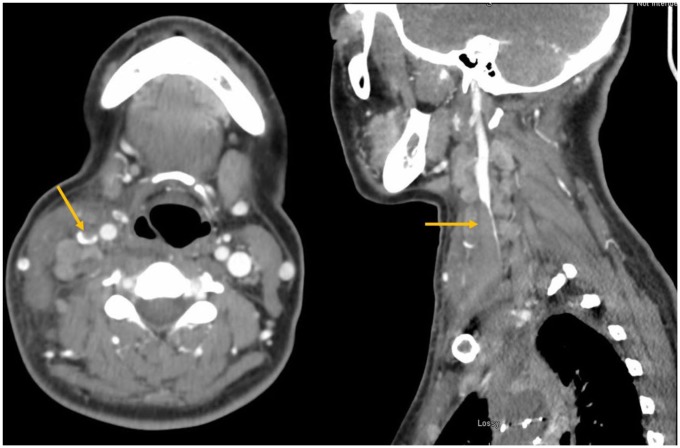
Computed tomography scan of the neck with intravenous contrast revealed
